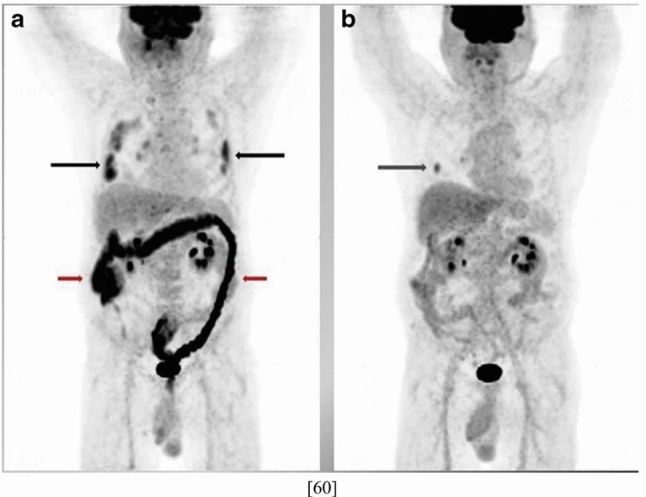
a The initial [18]F-labeled fluorodeoxyglucose PET/CT study reveals metabolically active lung infiltrates (black arrows), hypermetabolic hilar and mediastinal lymphadenopathy, and intense colonic activity (red arrows). b Follow-up PET/CT with resolution of lung and GI activity, leaving a metabolically active nodule that was obscured on the initial PET-CT by hypermetabolic COVID-19 pulmonary infiltrate (arrow). *CT* computed tomography, *GI* gastrointestinal, *PET* positron emission tomography (Reproduced with permission from Kidane et al. [59])
